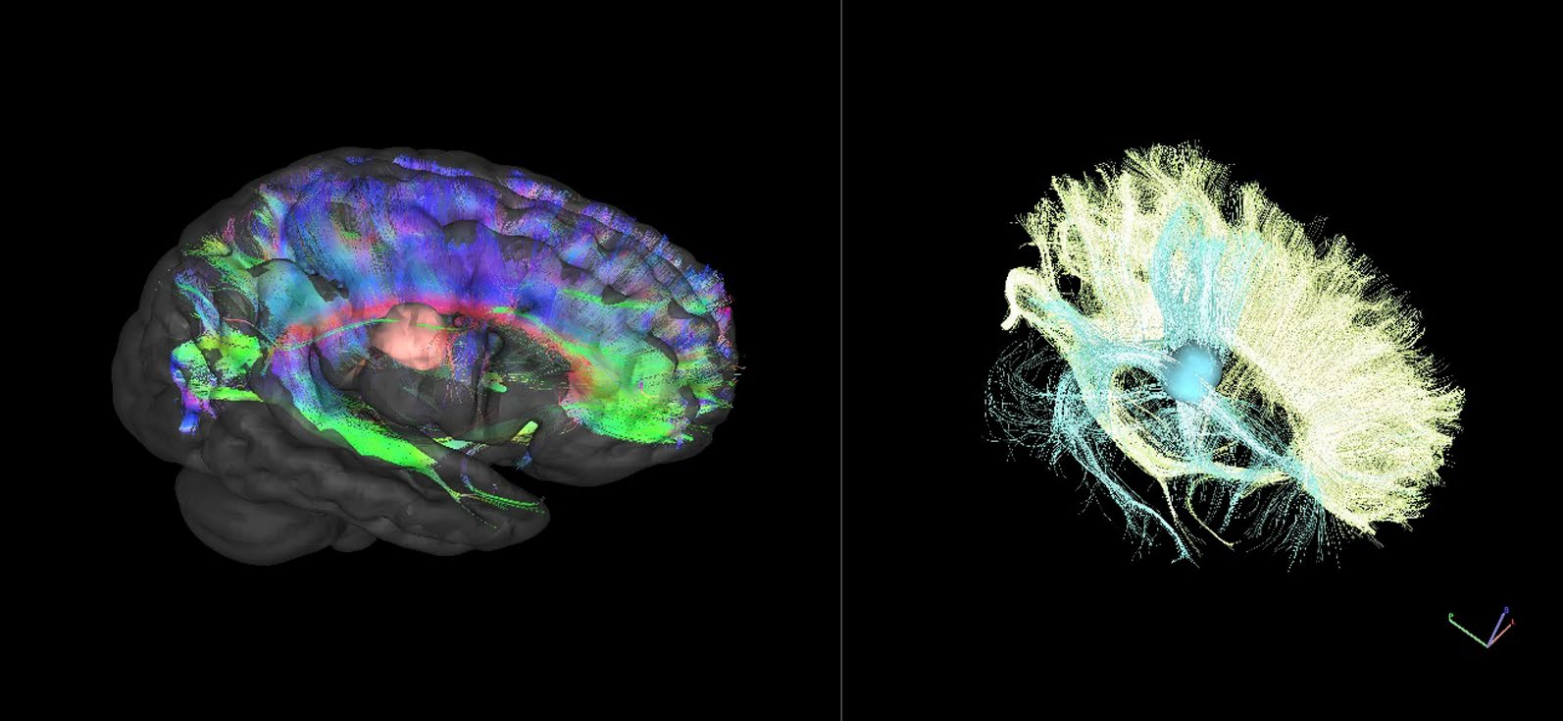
Example images of MRI fibre tracking. The stroke region is highlighted. Left: Colour-coded fibre tracks passing the midline corpus callosum. Right: Corpus callosum fibre tracks are shown in yellow, blue tracks were seeded from the stroke lesion
